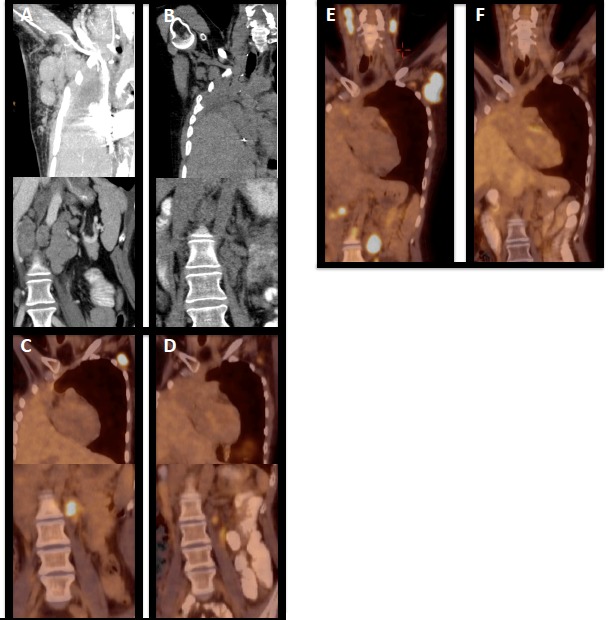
Course of disease under immunologic treatment (A) CT scan showing massively enlarged axillary and subdiaphragmal lymph nodes prior to the start of nivolumab, (B) CT control two months after start of nivolumab showing massive regression of nodes in both regions, (C) ^18^FDG PET/CT in progression during nivolumab and prior to start of ipilimumab (D) treatment response during ipilimumab (E) ^18^FDG PET/CT cervical, axillary , and subdiaphragmal lymph node progression after ipilimumab and prior to start of ruxolitinib (F) ^18^FDG PET showing ruxolitinib-induced remission.
